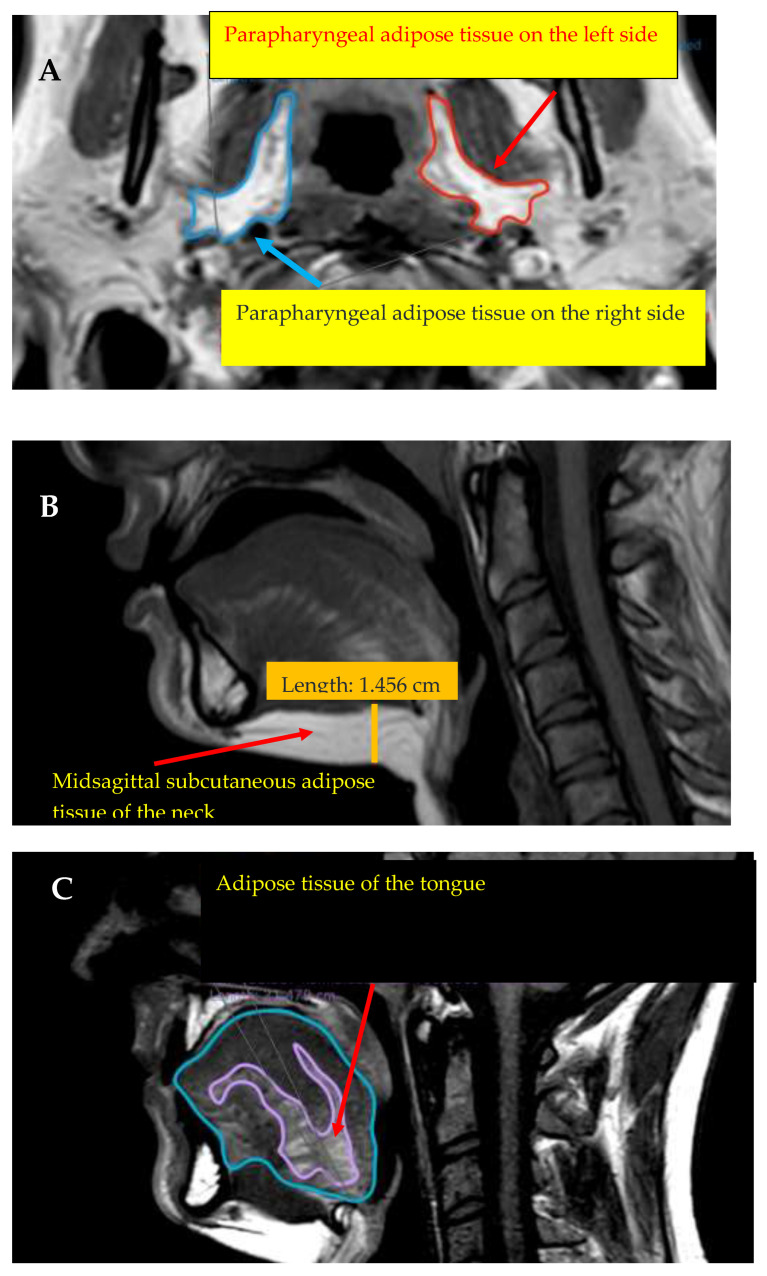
(A) T1-weighed measurements in the axial plane showing parapharyngeal adipose tissue on the left and right sides; (B,C) midsagittal axis of the T1-weighted MRI scans showing the midsagittal subcutaneous adipose tissue of the neck and adipose tissue of the tongue (taken from our data).
